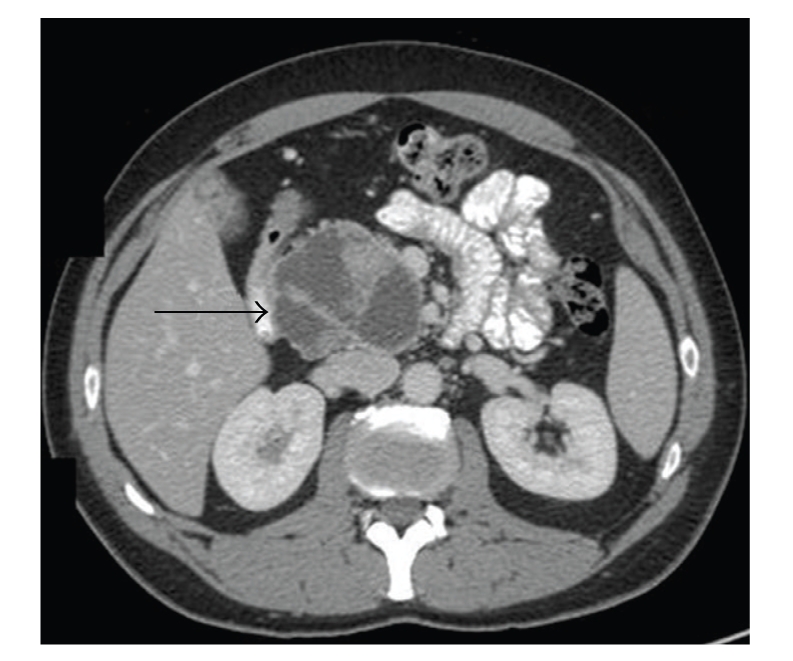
CT scan showed a large heterogeneous cystic lesion in the head of the pancreas (arrow).
